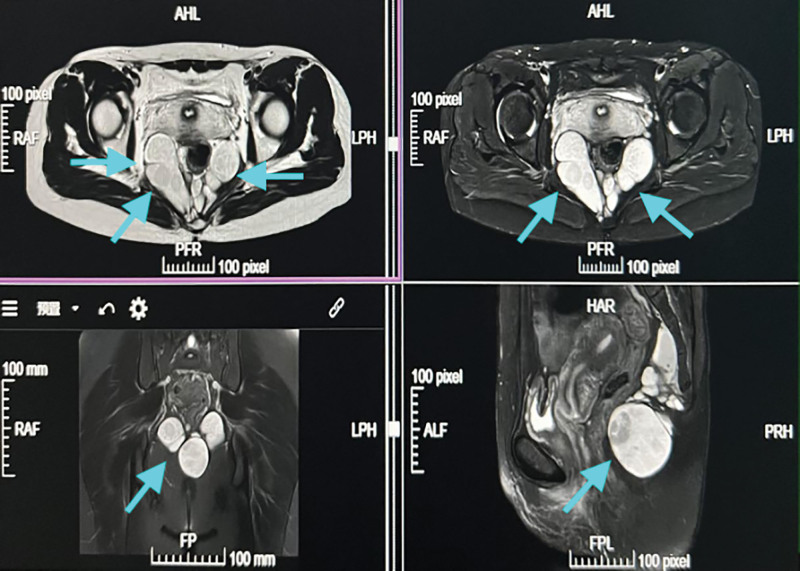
Pelvic MRI showed multiple sacrococcygeal teratomas in the sacrococcygeal region, the larger one was located 10,000 behind the rectoanal canal, fluid levels were observed in some lesions, and the rectum was compressed and moved anteriorly. MRI = magnetic resonance imaging.
